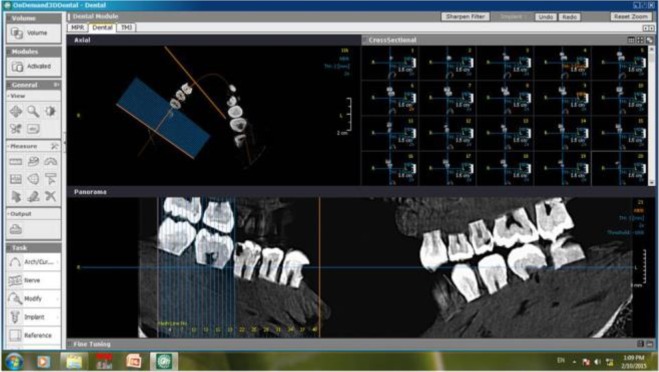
CBCT image of the skull holding the teeth
